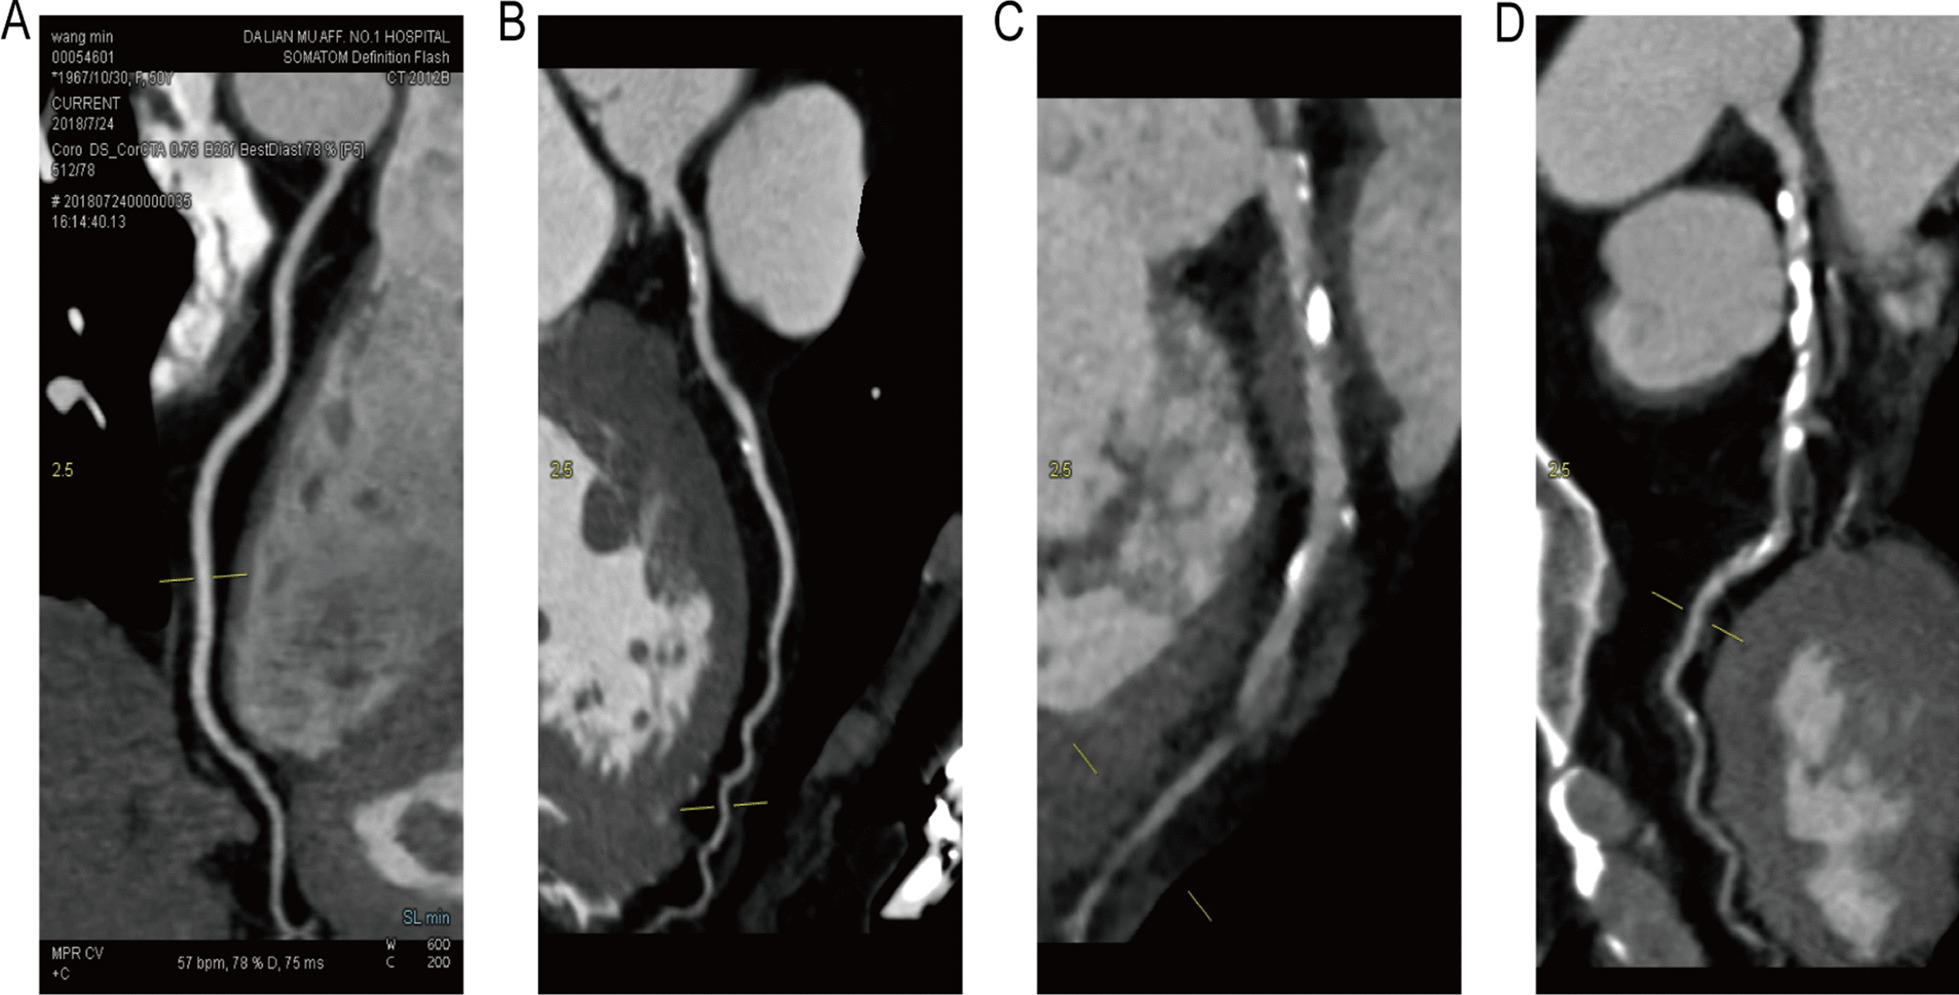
Representative images of CCTA of different levels of calcification. A Normal coronary without calcification. B Coronary with light calcification. C Coronary with moderate calcification. D Coronary with severe calcification. CCTA, coronary computed tomography angiography
